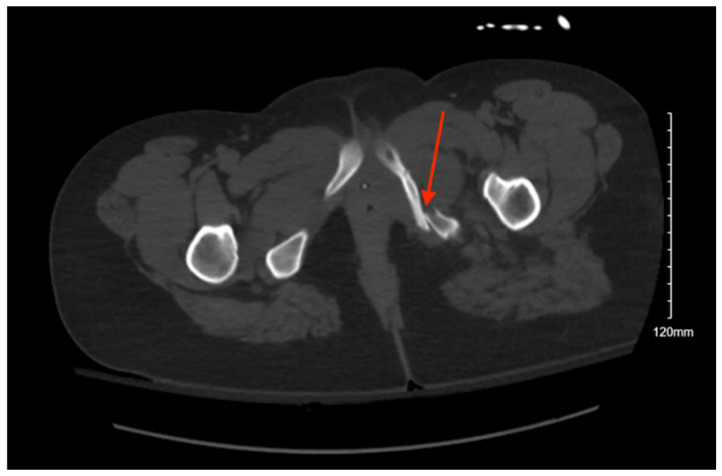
CT abdomen/pelvis images of the described fractures in case 13. Red arrows are used to indicate the location of pelvic fractures for easier identification.
